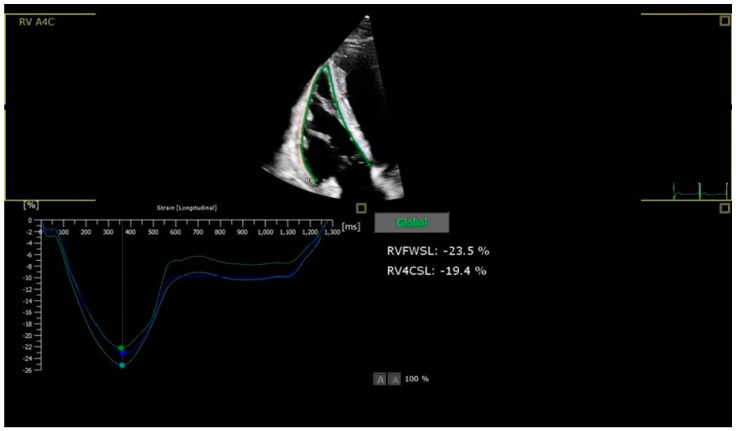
Strain echocardiography RV-FWSL and RV-4CSL images.
